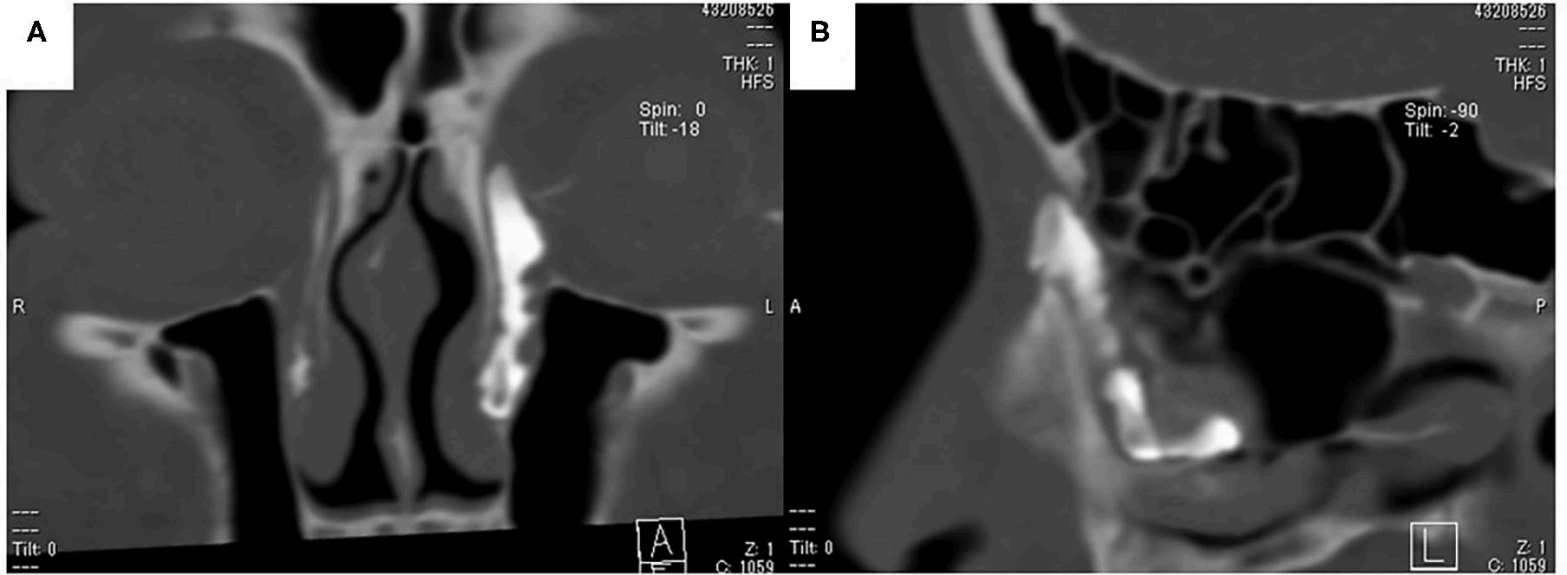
CT-dacrocystographic findings. (A) Coronal view: contrast medium was vertically located from the lacrimal sac to the orifice. (B) Sagittal view: contrast medium accumulated posteriorly from the lacrimal orifice.
